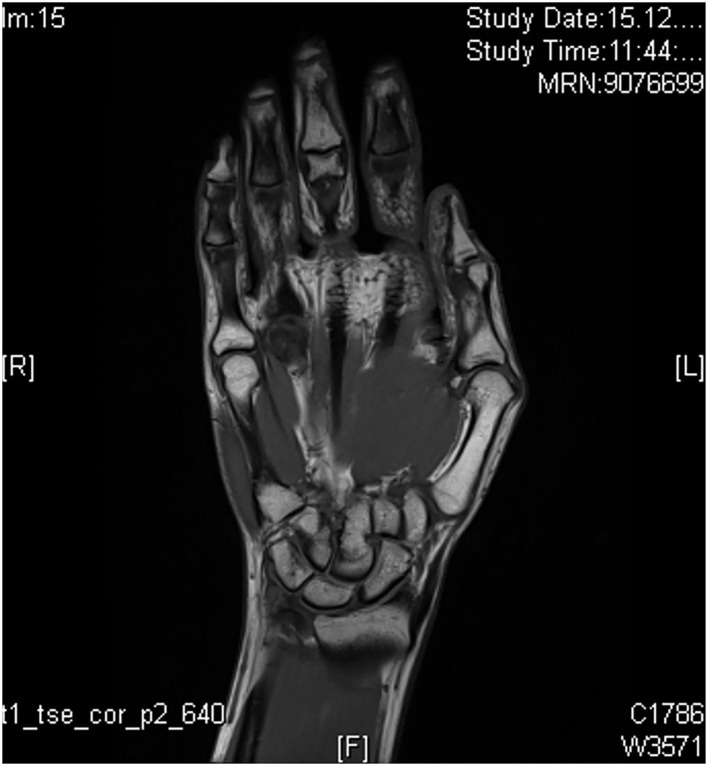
MRI of the left hand without signs of inflammation (T1 sequence).
